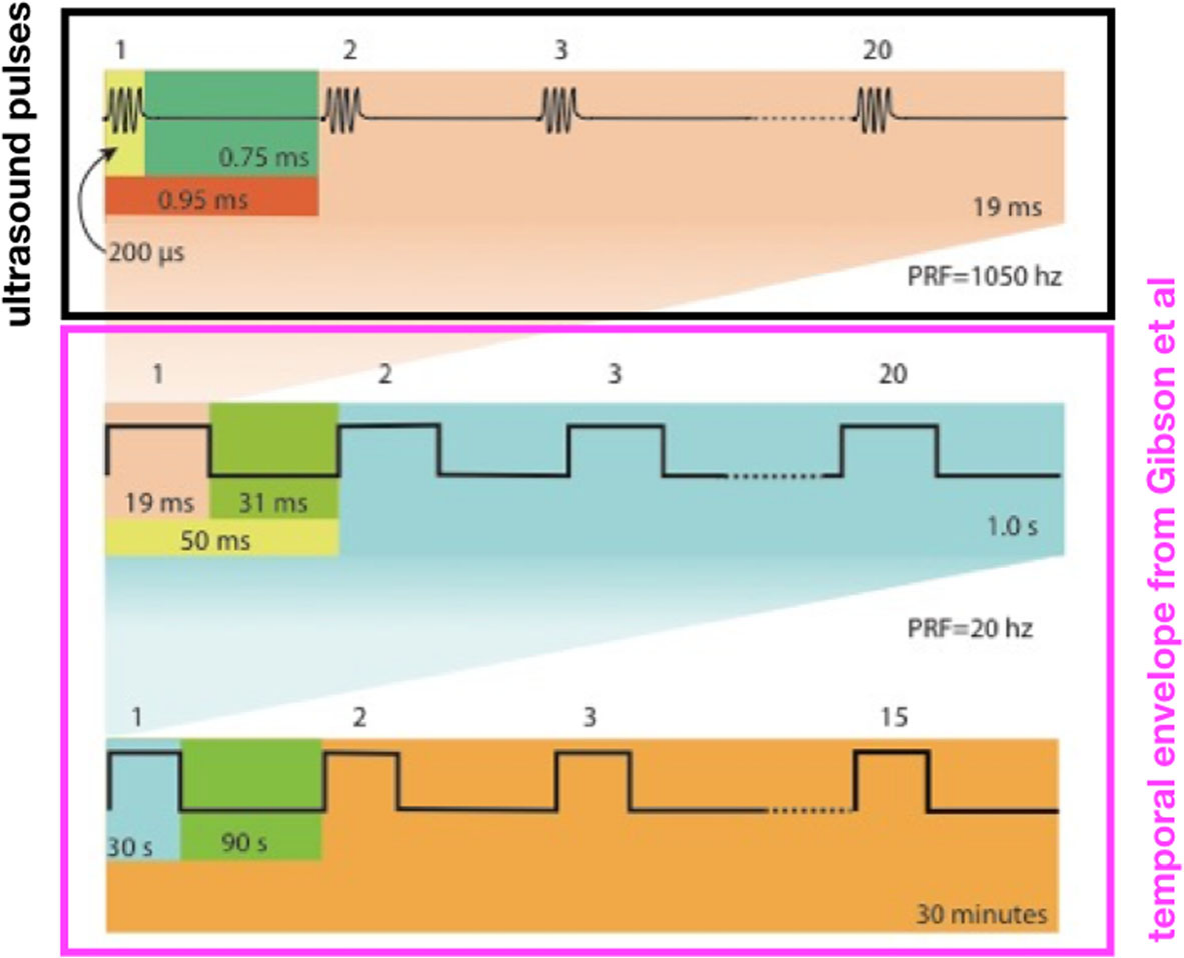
Temporal pattern of ultrasound delivery, modeled after the optical stimulation pattern of Gibson et al. The optical protocol delivered light twenty times per second, with each pulse of light lasting 25 milliseconds (ms), for a total of 30 s. Each 30-s long period of pulsed stimulation preceded a 90-s long period of no stimulation. These on-off periods of light exposure lasted a total of 30 min per day, applied for five contiguous days. We modified this protocol to application of ultrasound by using *pulsed* ultrasound during *each* of 19-ms long time periods, followed by 31 ms of no ultrasound, rather than *continual* light for 25 ms followed by 25 ms of no light. The figure here shows 20 ultrasound pulses applied during each 19 ms time period, each pulse lasting 200 microseconds
